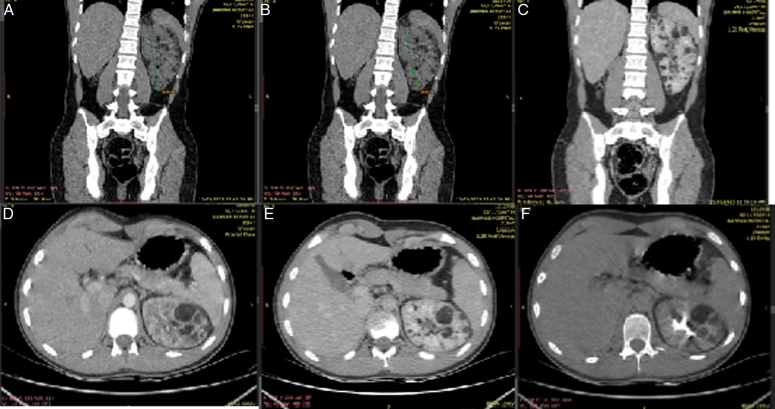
Several views of the abdomino-pelvic computed tomography scan showing a single left kidney presenting with angiolipomatosis [(A, B) being plain coronal without contrast showing enlarged kidney dimensions with a longitudinal diameter of d=16.46 cm and no corticomedullary differentiation, (C) being in arterial phase, (D) in portal phase, and (E, F) in delay phase].
